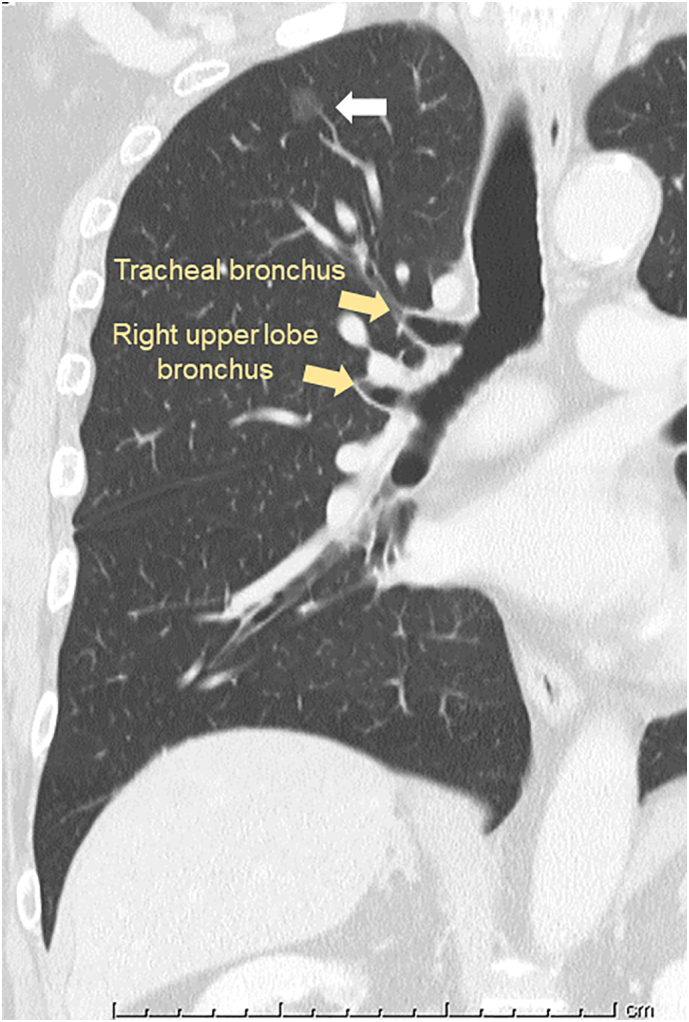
Preoperative coronal computed tomography image. A pure ground-glass opacity nodule (white arrow) is located in the right apical segment that is supplied by a tracheal bronchus.
